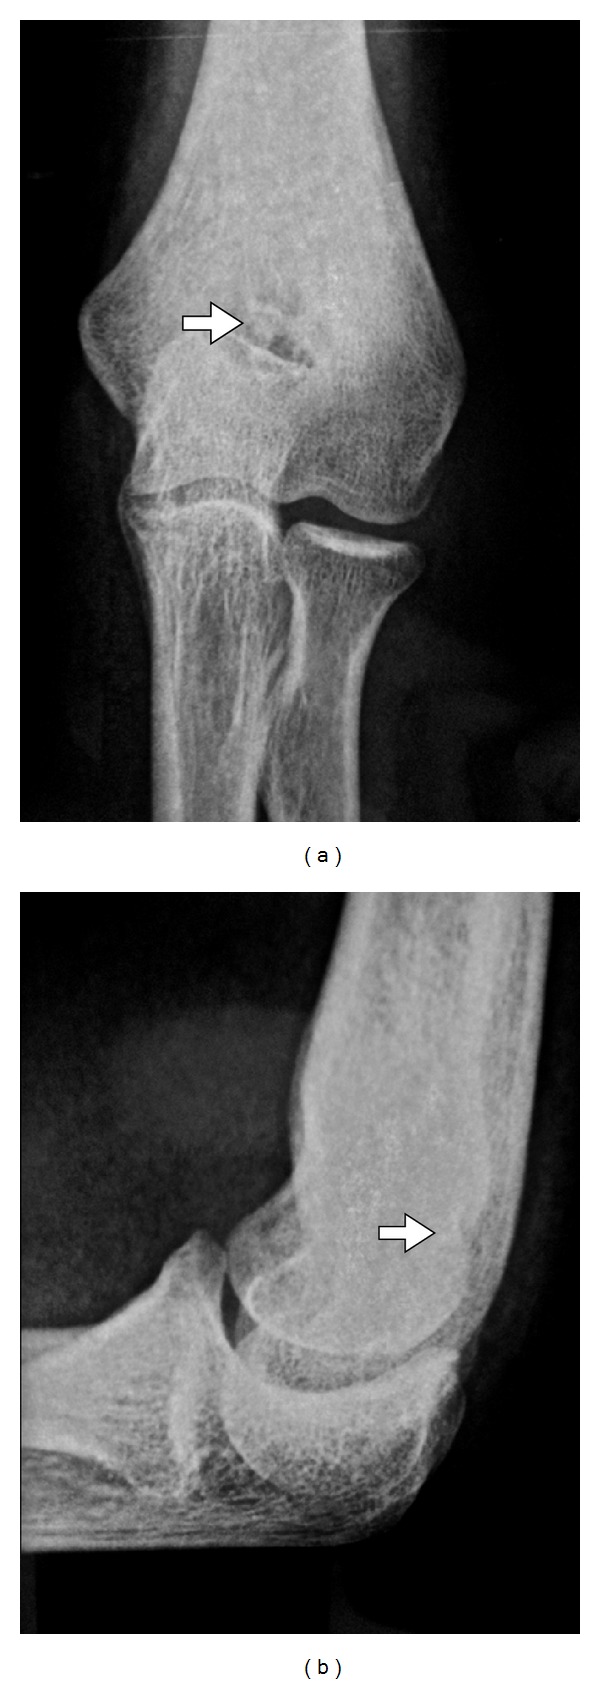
AP/lateral plain radiograph: a nidus in olecranon fossa humeri with small central sclerosis surrounded by reactive sclerosis.
